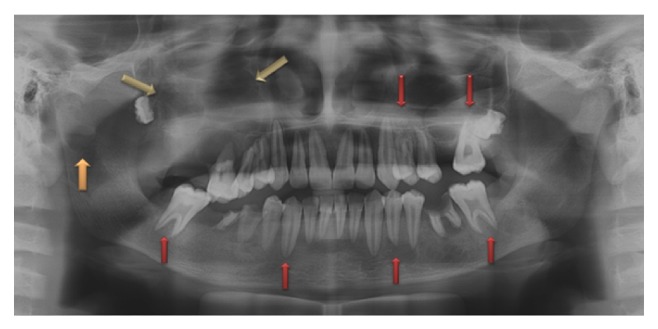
Preoperative radiograph represents multilocular radiolucency of maxilla, generalised loss of lamina dura, and unilocular radiolucency in condylar region.
